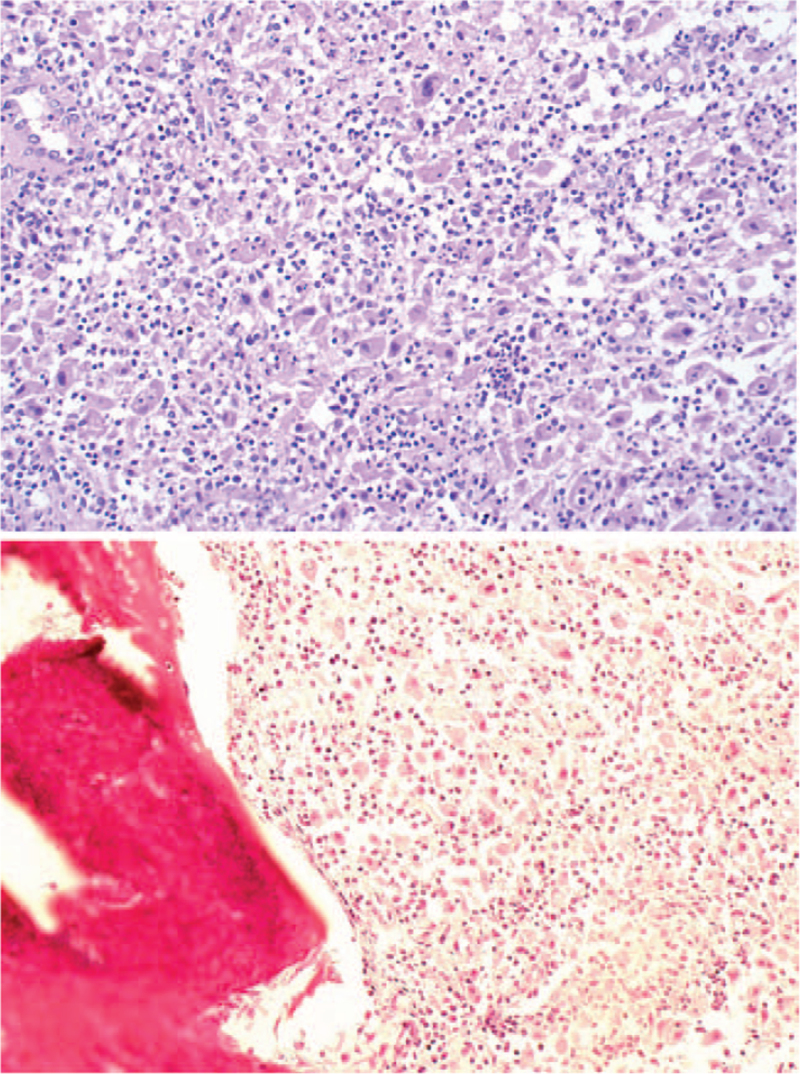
Postoperative CT images. The postoperative appearance of the L3 vertebral spinous process was irregular with a slightly high density and local subcutaneous strip shadow.
